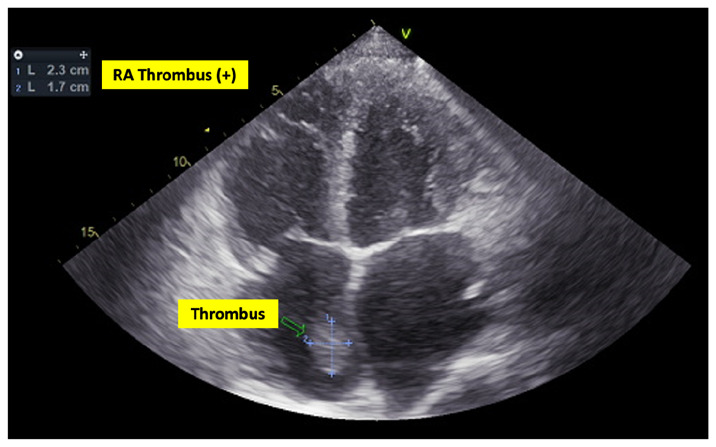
Transthoracic echocardiogram (TTE) showing thrombus in the right atrium. RA = right atrium.
